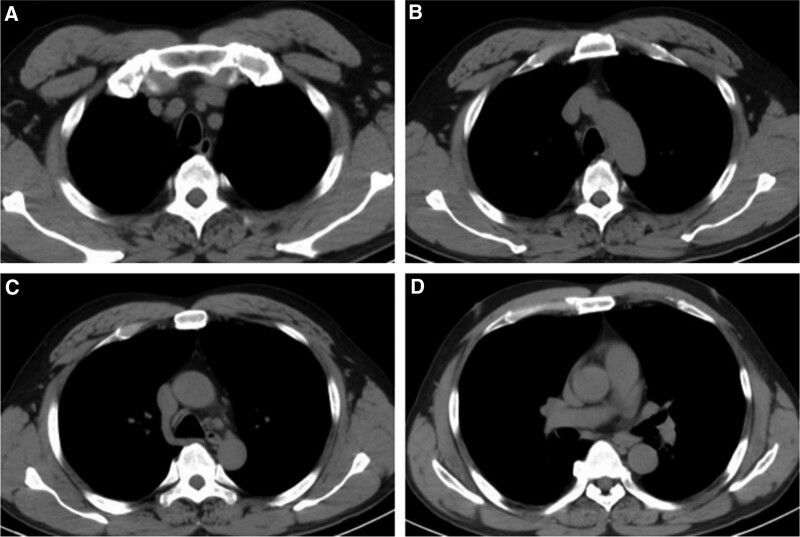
Computed tomography before the administration of immune treatment showing slight enlargement of mediastinal and bilateral hilar lymph nodes.
